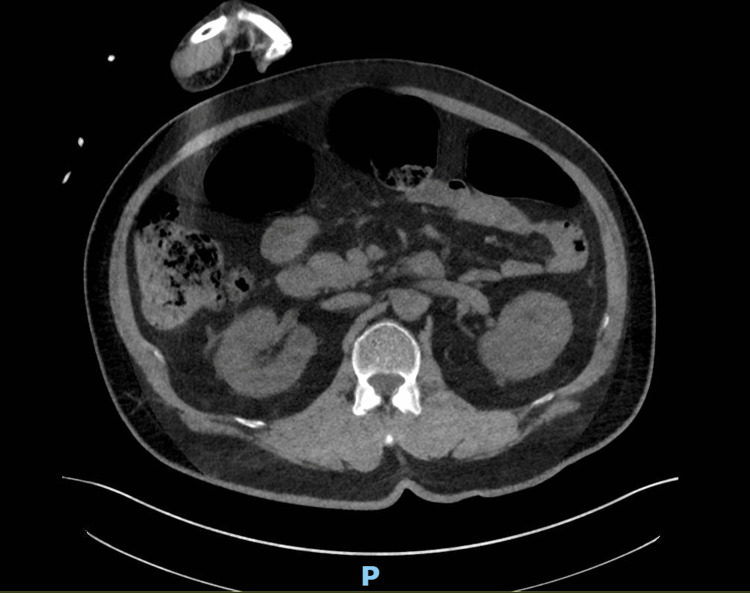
Contrast-enhanced CT of the thorax, abdomen, and pelvis (CT TAP). No evidence of malignancy or source of infection in the abdominal region.
